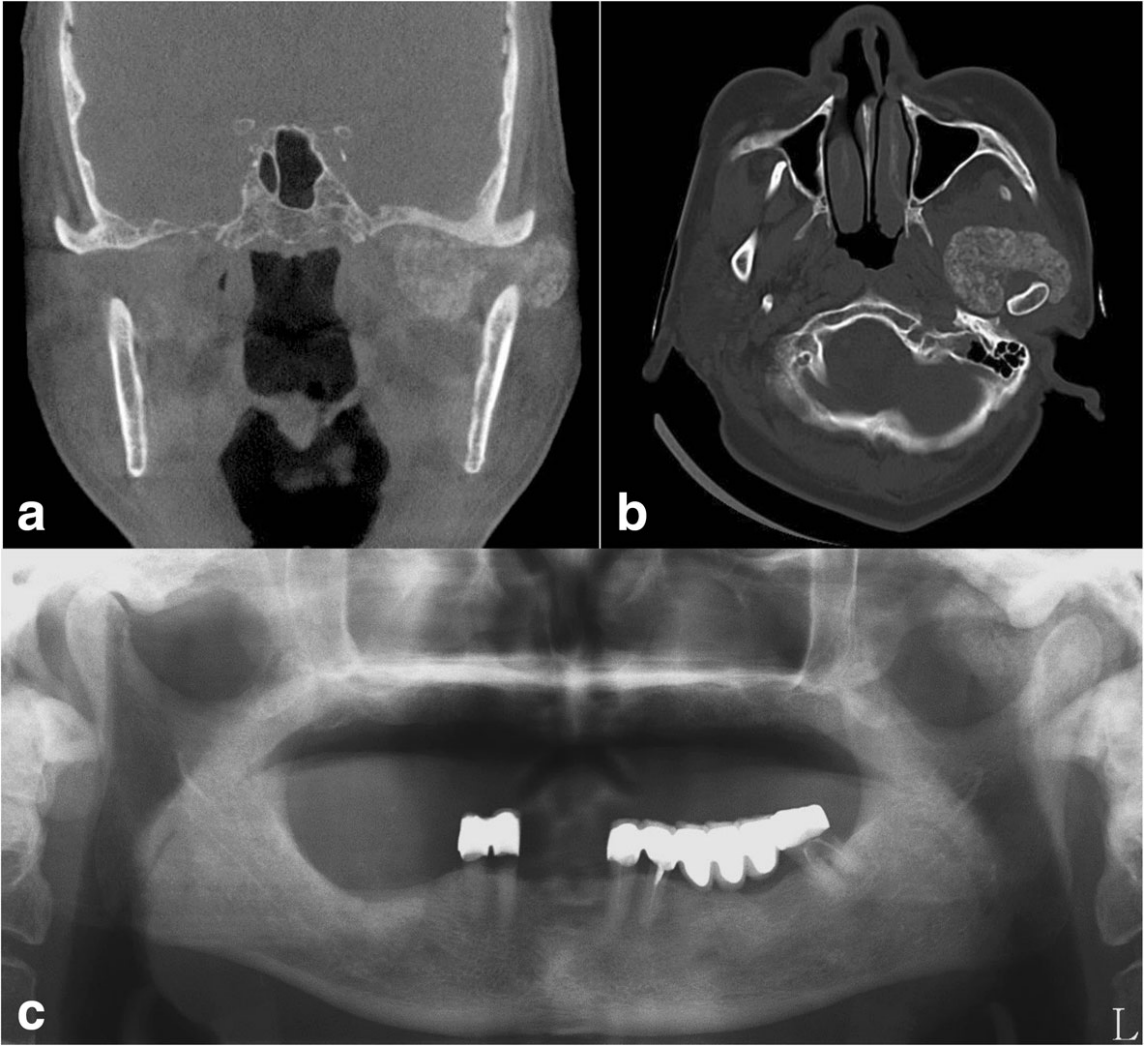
A well-defined calcified mass in the left temporomandibular joint space and infratemporal fossa. a Coronal view and b axial view of CBCT and c preoperative panoramic view
